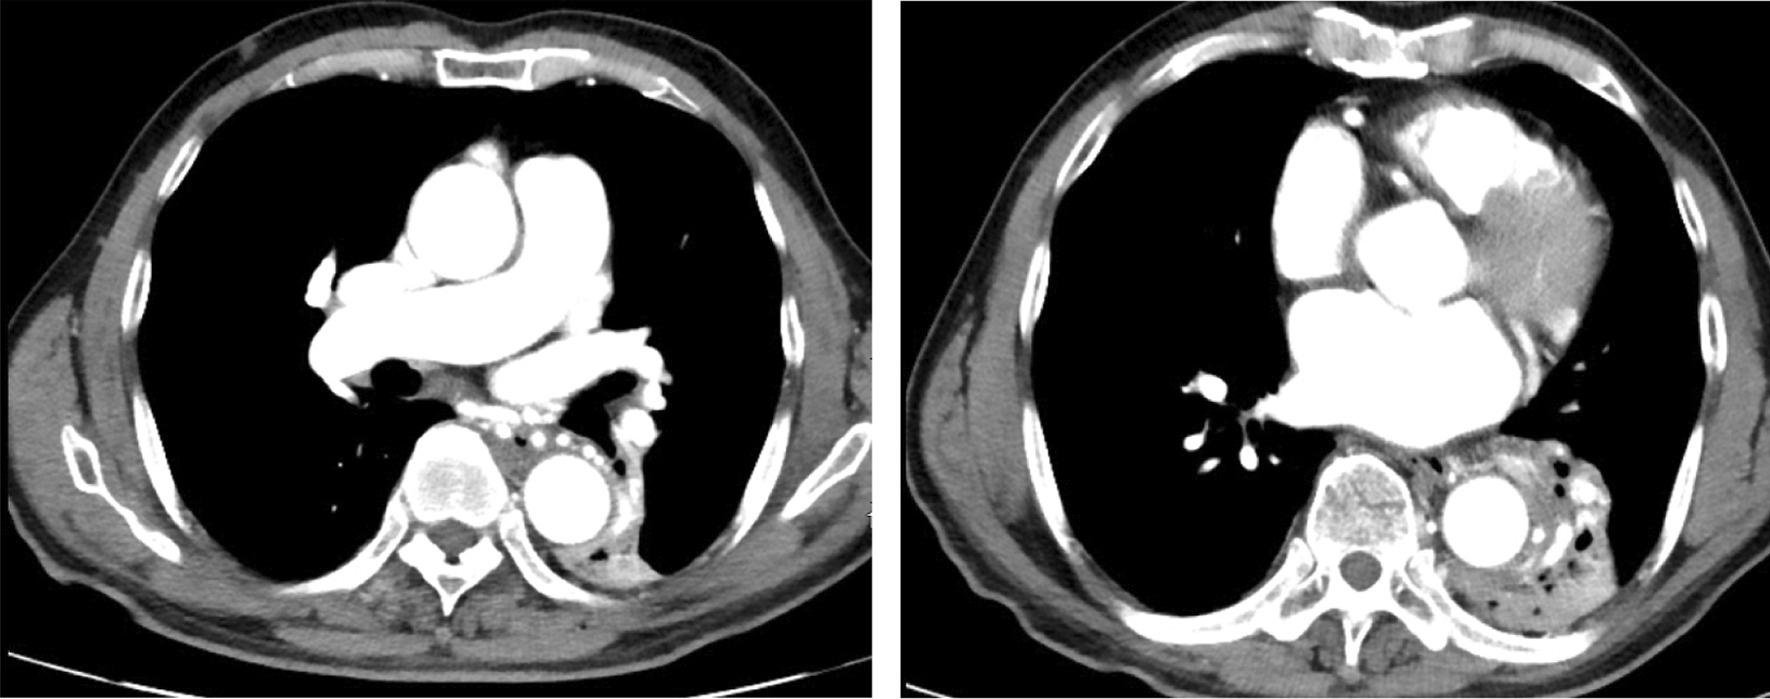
Computed tomography angiophy of bronchial artery (cross section): the left bronchial artery branches are tortuous and dilated, the volume of the left lower lobe of the lung shrinks, and the lung tissue consolidation shows soft tissue density shadow
